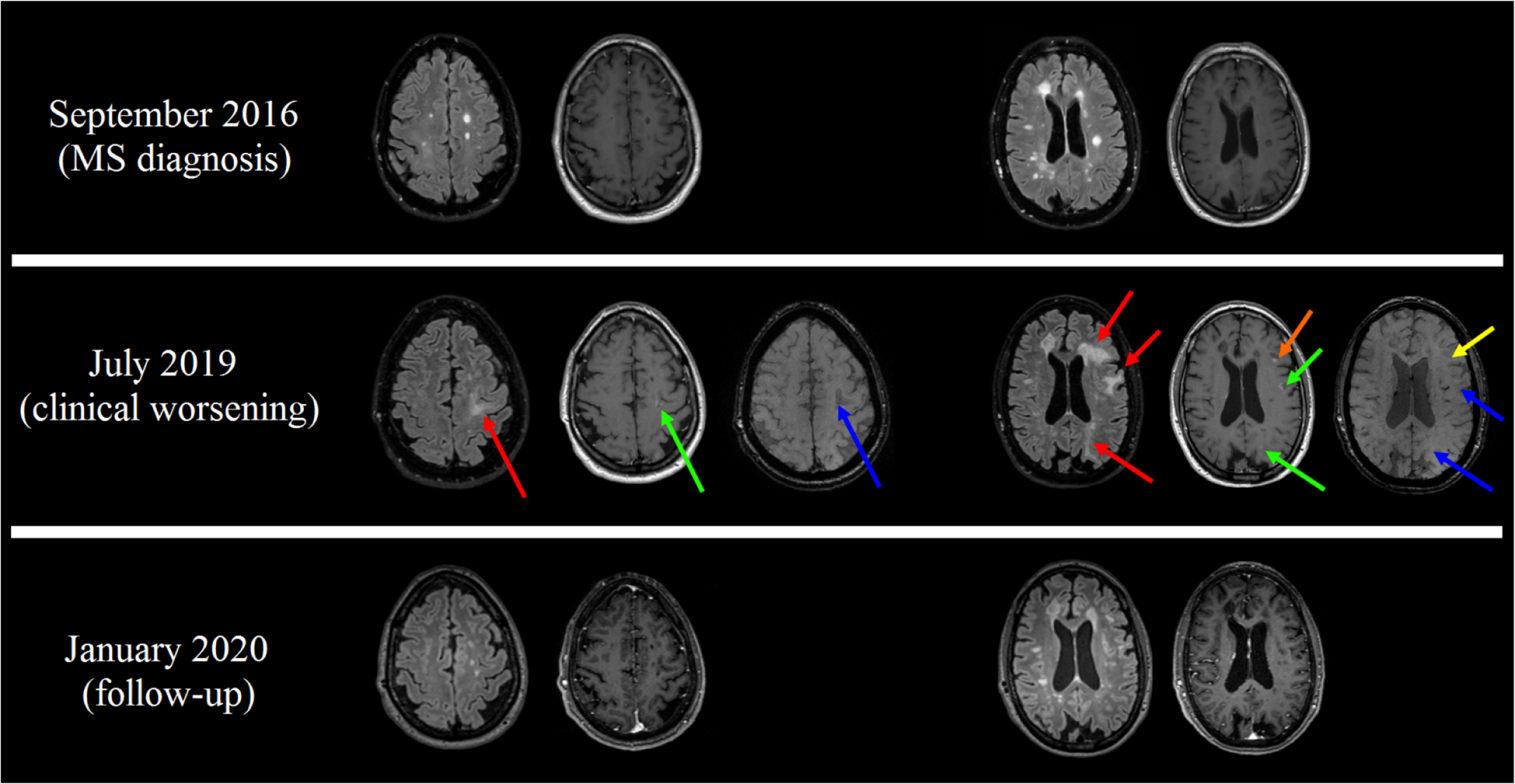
Selected MRI findings in our Multiple Sclerosis (MS) case. At the baseline exam (September 2016, upper row) a typical radiological MS pattern was present. When the patient presented a subacute clinical worsening (July 2019, middle row), brain MRI scan showed the presence of four new confluent Fluid Attenuated Inversion Recovery (FLAIR) hyperintense lesions (red arrows), with involvement of the U-fibers, a sharp border between the superficial aspect of the lesion and the overlying cortex and an ill-defined deeper border. Three of these lesions showed a punctate peripheral contrast-enhancement (green arrows) and a mild, but consistent, hypointense subcortical rim in Spoiled Gradient Recalled (SPGR) images (blue arrows), suggestive of PML lesions. Along with these lesions, a left frontal oval-shaped lesion showed a different pattern of contrast enhancement compared to the other lesions (orange arrow), in absence of hypointense signal in SPGR images (yellow arrow), more suggestive of active MS rather than a PML lesion. At a follow-up examination (January 2020, lower row), the PML lesions showed an almost complete resolution, in absence of significant sequelae, while the oval-shaped FLAIR hyperintense lesion was still present, although reduced in volume
